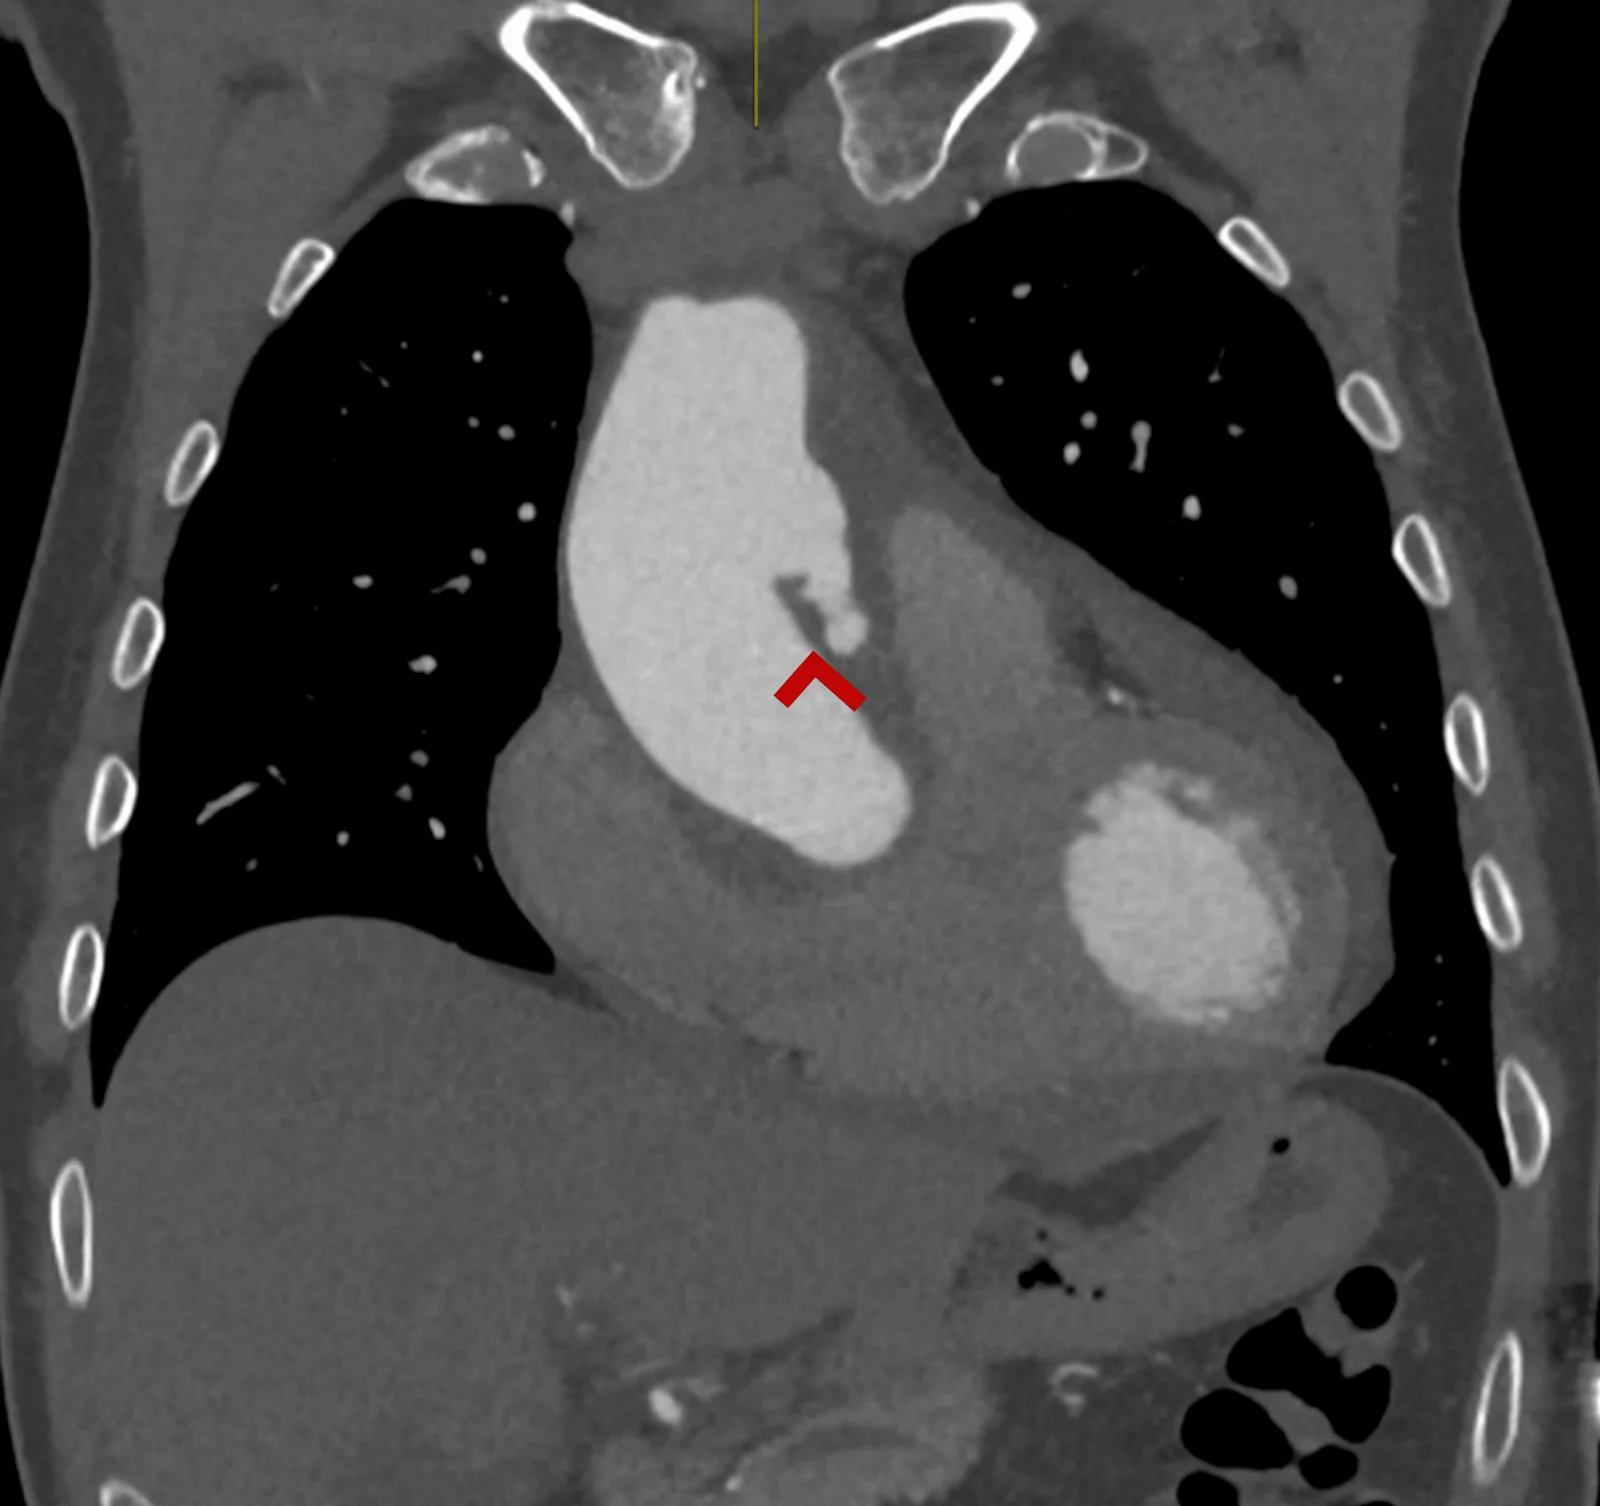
CT scan, coronal multiplanar reformat. The red arrow indicates outpouching of the ascending aorta.
